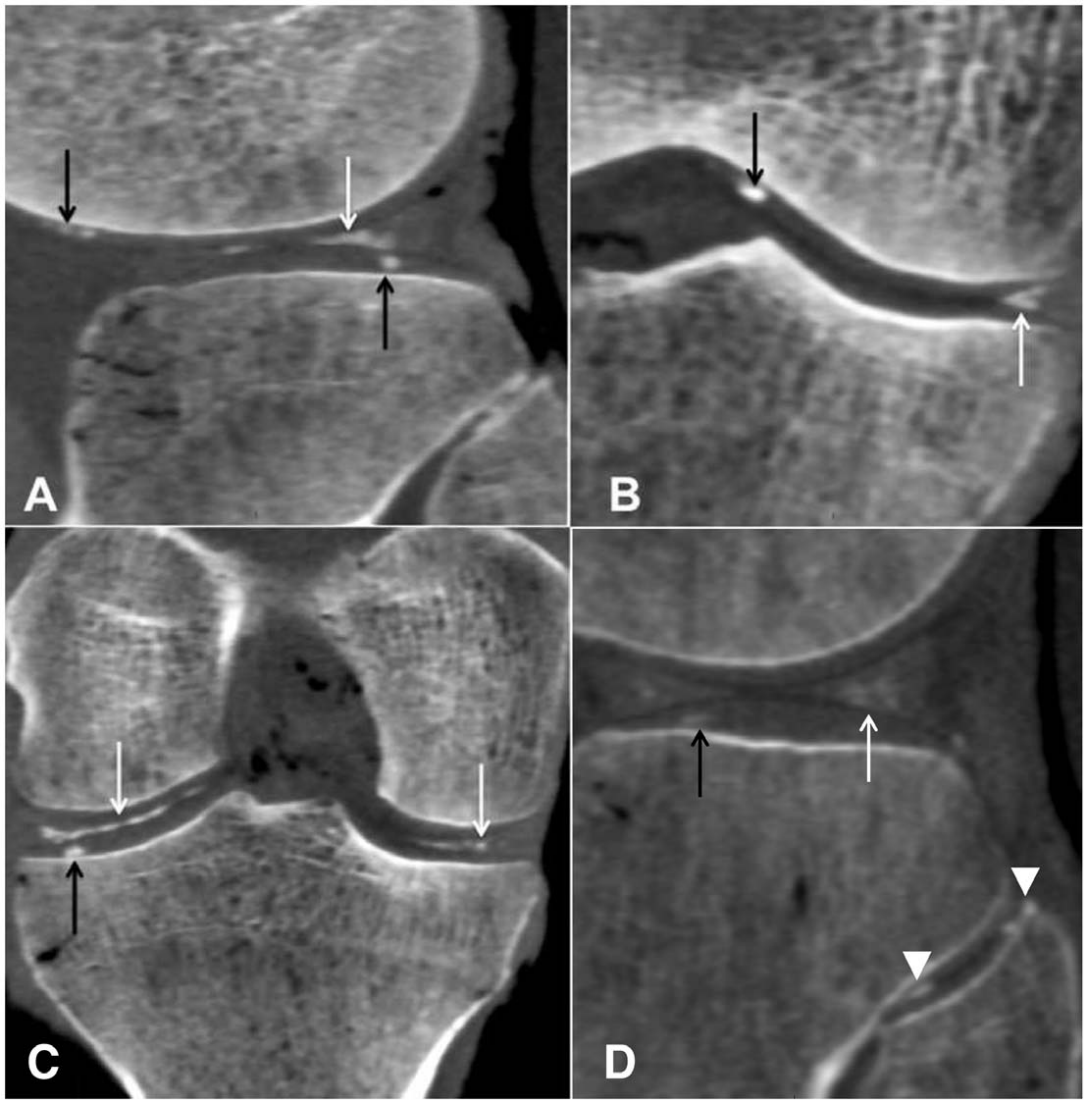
Computed tomography scans of the same knee demonstrating calcifications within the hyaline cartilage (black arrows, arrow heads) and the meniscal segments (white arrows) in the femoro-tibial joint on a sagittal (A) and coronal (B–C) reformations, and in the proximal tibio-fibular joint on a sagittal reformation (D).
